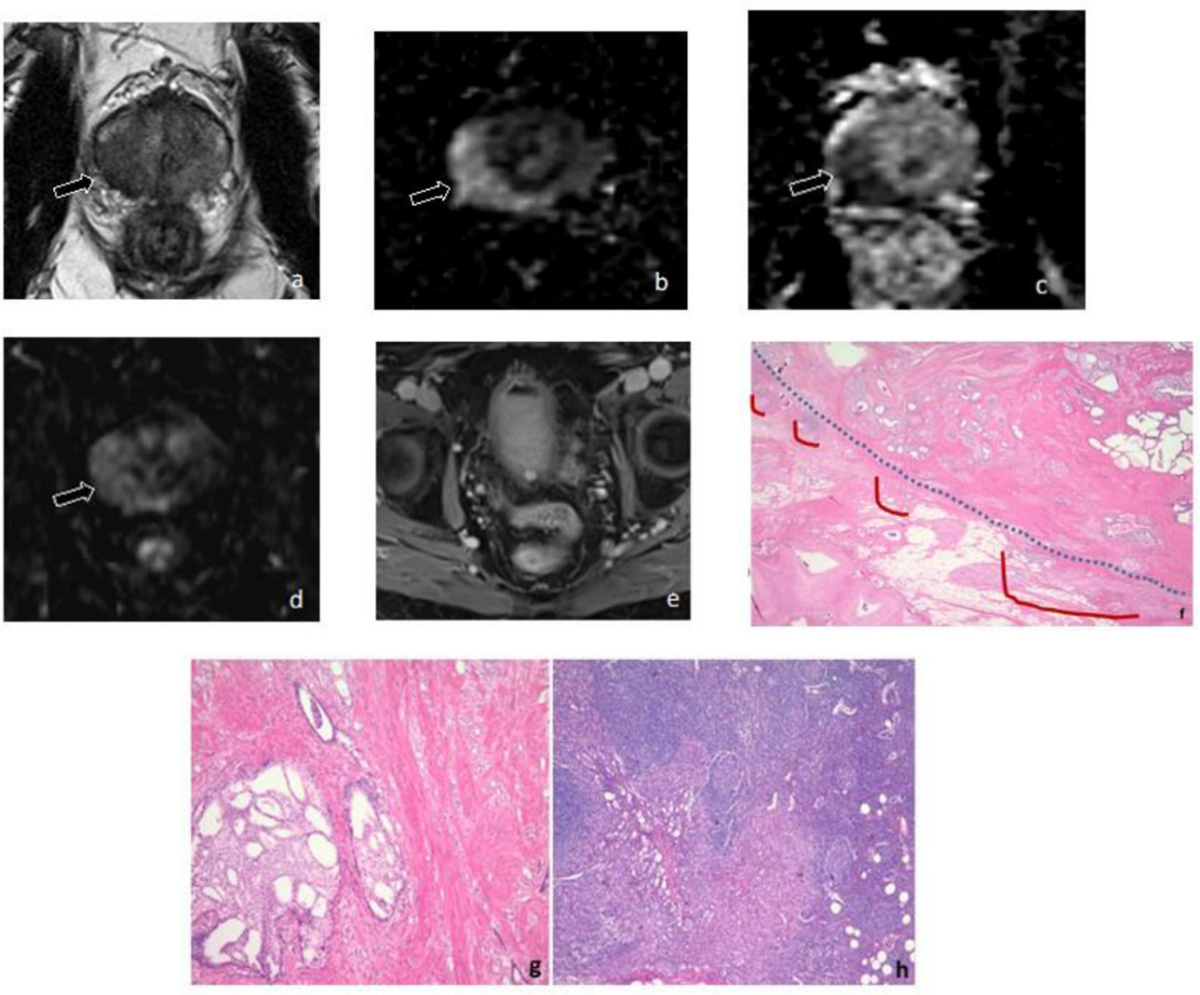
Patient with MRI-invisible PLNM. A 66-year-old man had a prostate-specific antigen level of 2.09 ng/mL and ISUP-GG 2 prostate cancer on biopsy. (A) Axial *T*
